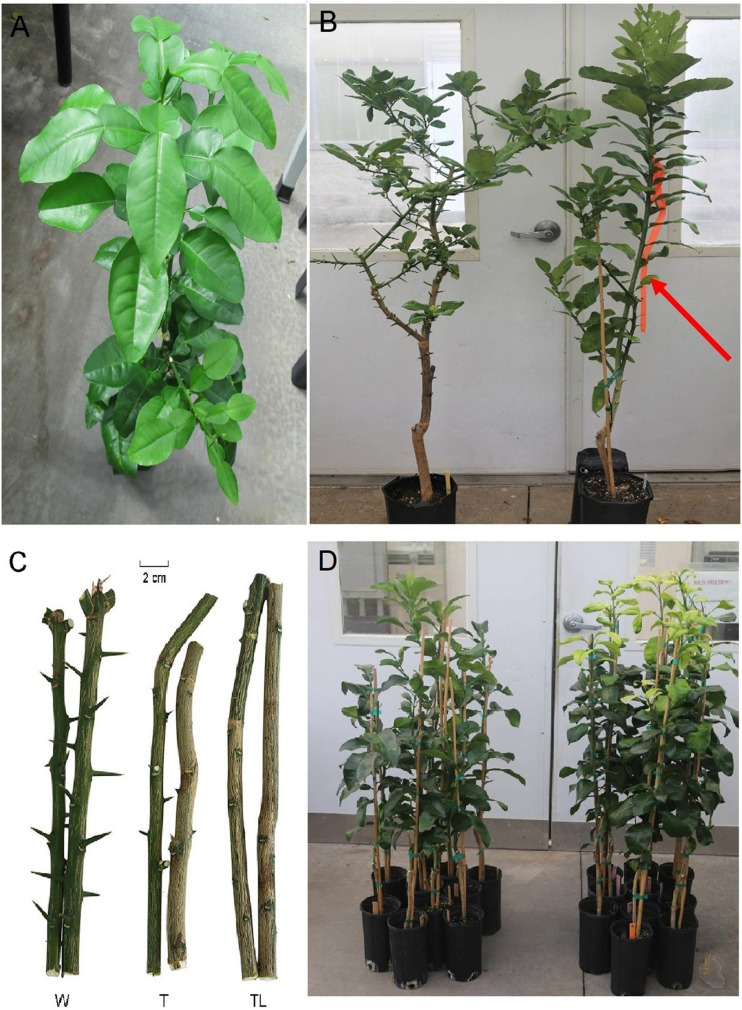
Pummelo thornless bud-sport selection and evaluation. (A) Asymptomatic HLB-tolerant Mato Buntan seedling (wild type W) with CT = 24.5 1 year after Las inoculation. (B) Comparison of the growth of W (left) and thornless bud-sport (red arrow). (C) The branches of W, T, and TL. (D) The HLB-tolerant mutants (TL and T) in the left show growth retardation, while the susceptible grapefruit plants on the right showed typical yellow shoot symptoms 12 months after inoculation with an aggressive Las isolate.
